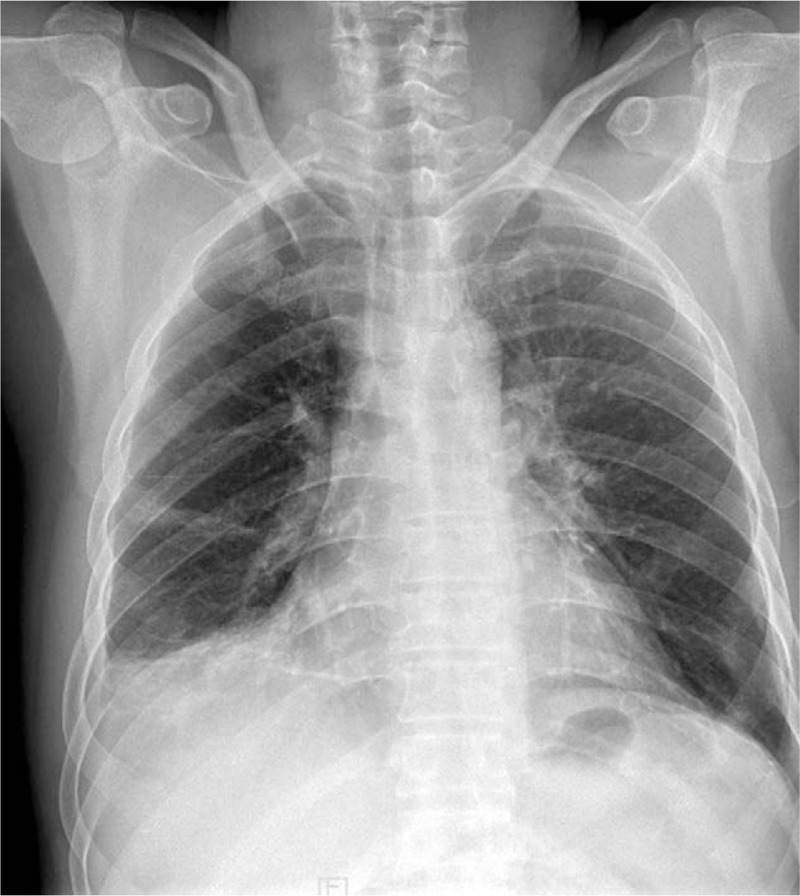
Chest radiograph revealed infiltration shadow in lower part of both lungs.
